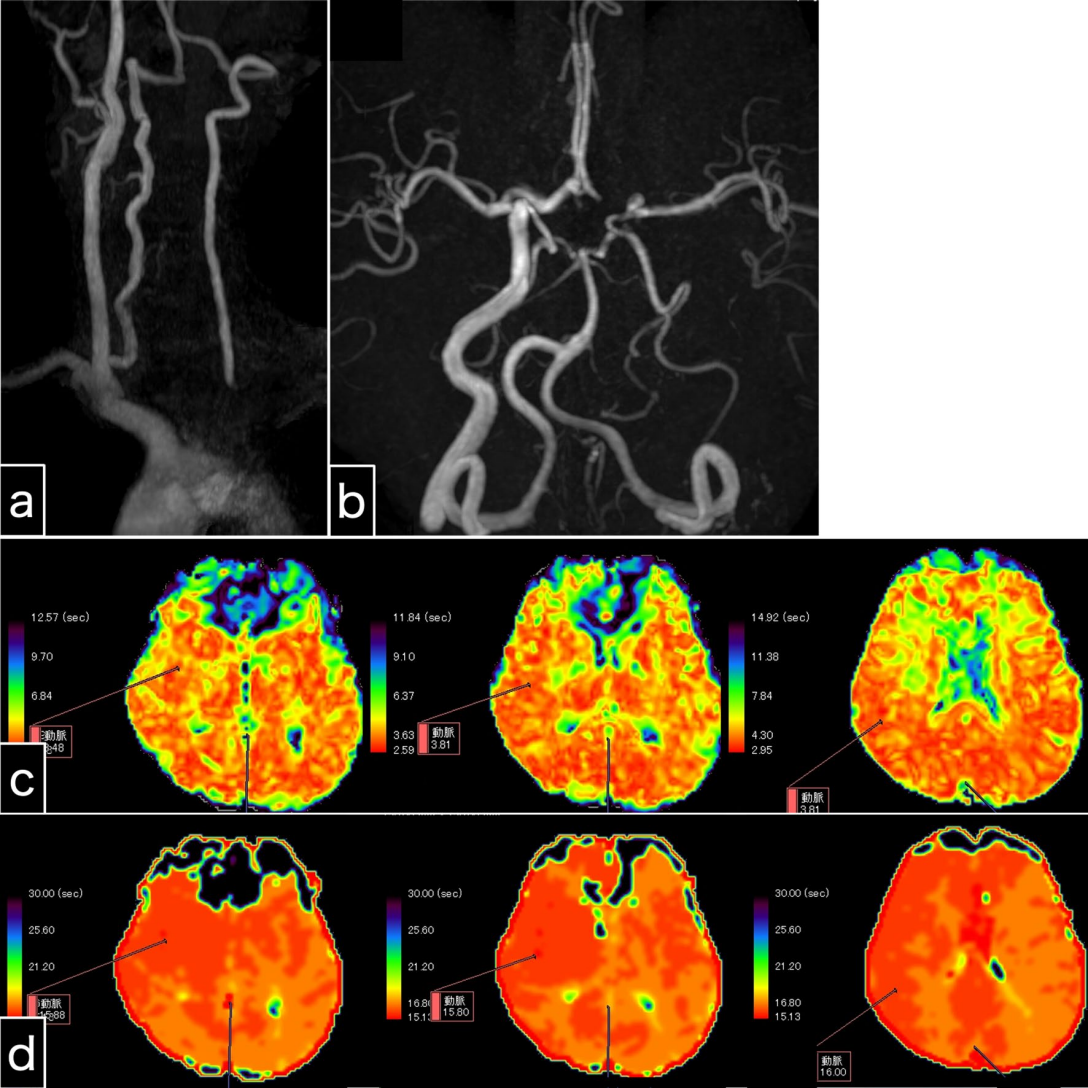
Examination 1 day after left subclavian artery stenting. a Cervical magnetic resonance angiography shows potent antegrade left vertebral artery flow. b Intracranial magnetic resonance angiography shows that the left vertebral artery intensity is stronger than that of the pre-stenting magnetic resonance angiography. The collateral blood flow via the left posterior communicating artery, and not the anterior communicating artery, is depicted. c, d Perfusion magnetic resonance imaging. Maps of the mean transit time (c) and time to peak (d) reveal slightly prolonged times in the left cerebral hemisphere. Perfusion magnetic resonance imaging seems to show reduced laterality
